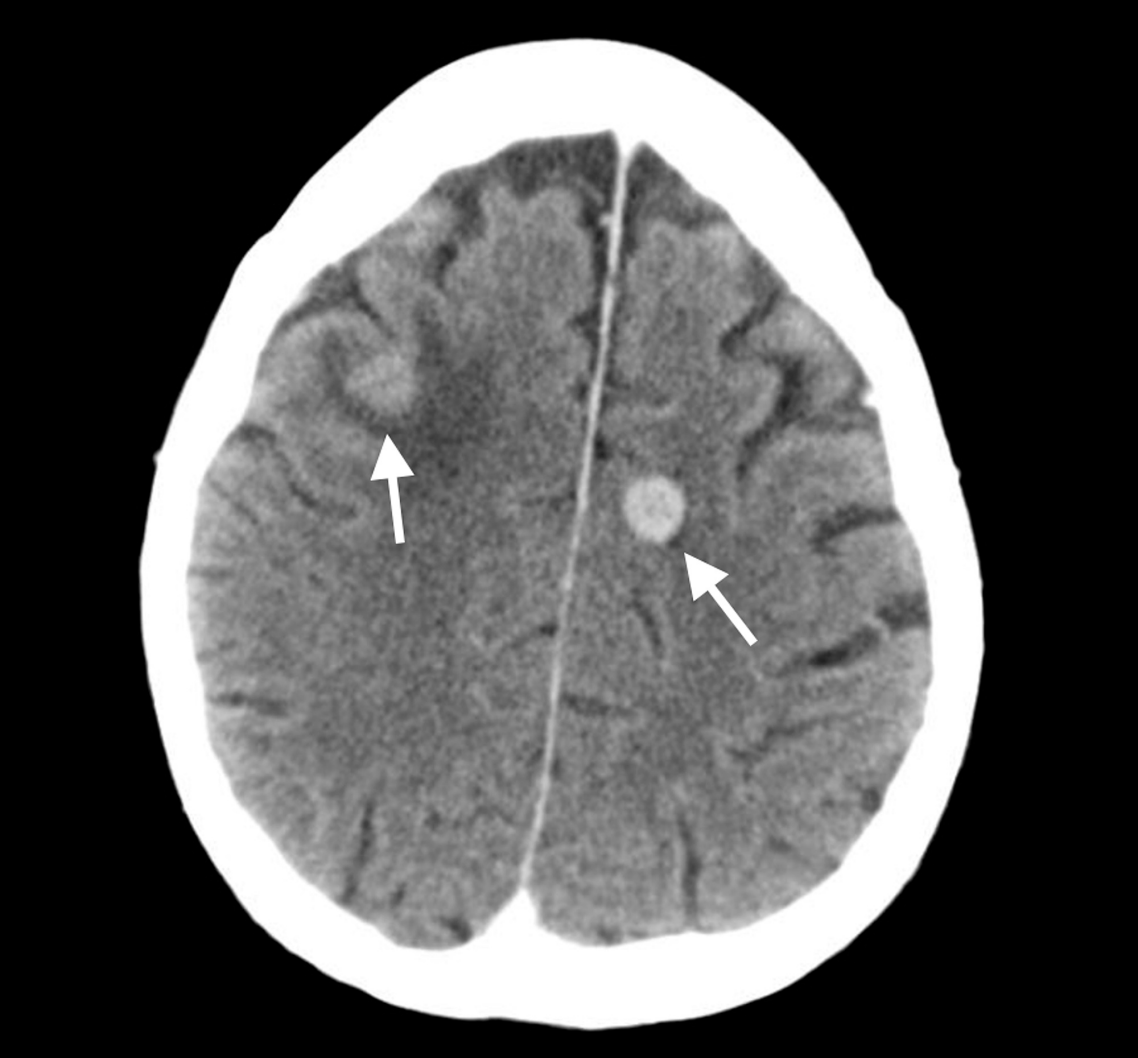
CT of the head showing multi-focal enhancing masses with surrounding edema
